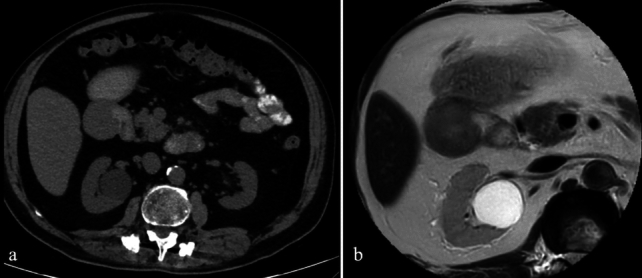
a. Non-enhanced axial CT image, b. Axial T2-weighted MRI image of a 62-year-old male patient, showing a round, well-defined, homogeneous lesion diagnosed as leiomyoma
